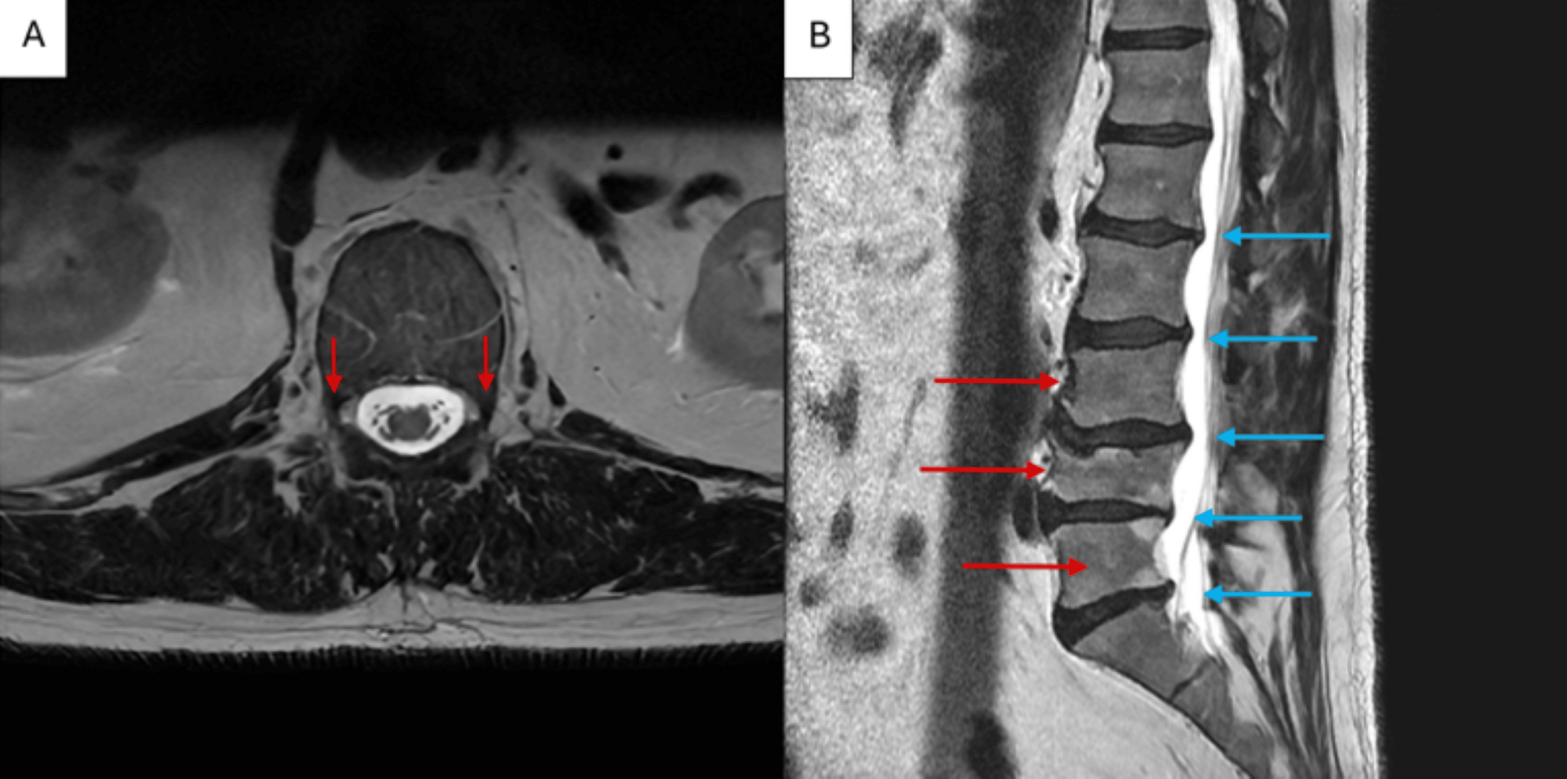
MRI of the lumbar spine. (A) T2-weighted axial view showing right- and left-sided neural foraminal narrowing. (B) T2-weighted sagittal view showing multilevel degenerative changes (red) and mild canal stenosis (blue)
